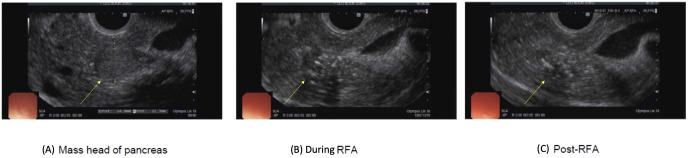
Images taken during endoscopic ultrasound (EUS) and radiofrequency ablation (RFA) showing (A) a hypoechoic lesion in the head of the pancreas. EUS image of the lesion (B) during the RFA and (C) after the RFA. A total of 4 ablations (30W of 10 seconds each) were conducted into the tumor.
